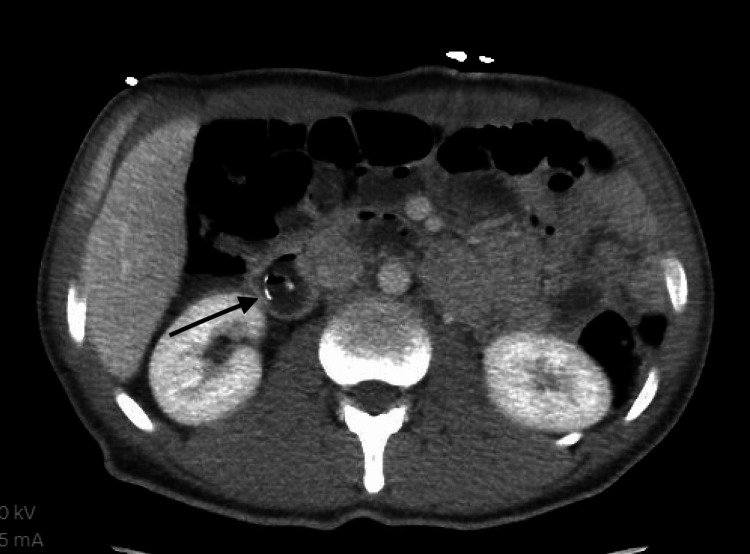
CT abdomen with contrast obtained six years prior with a finding of an unusual radiopaque tubular foreign body extending from the duodenum vertically into the colon
